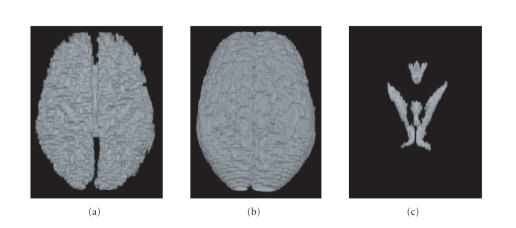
Segmentation of one MRI data set. Three-dimensional
